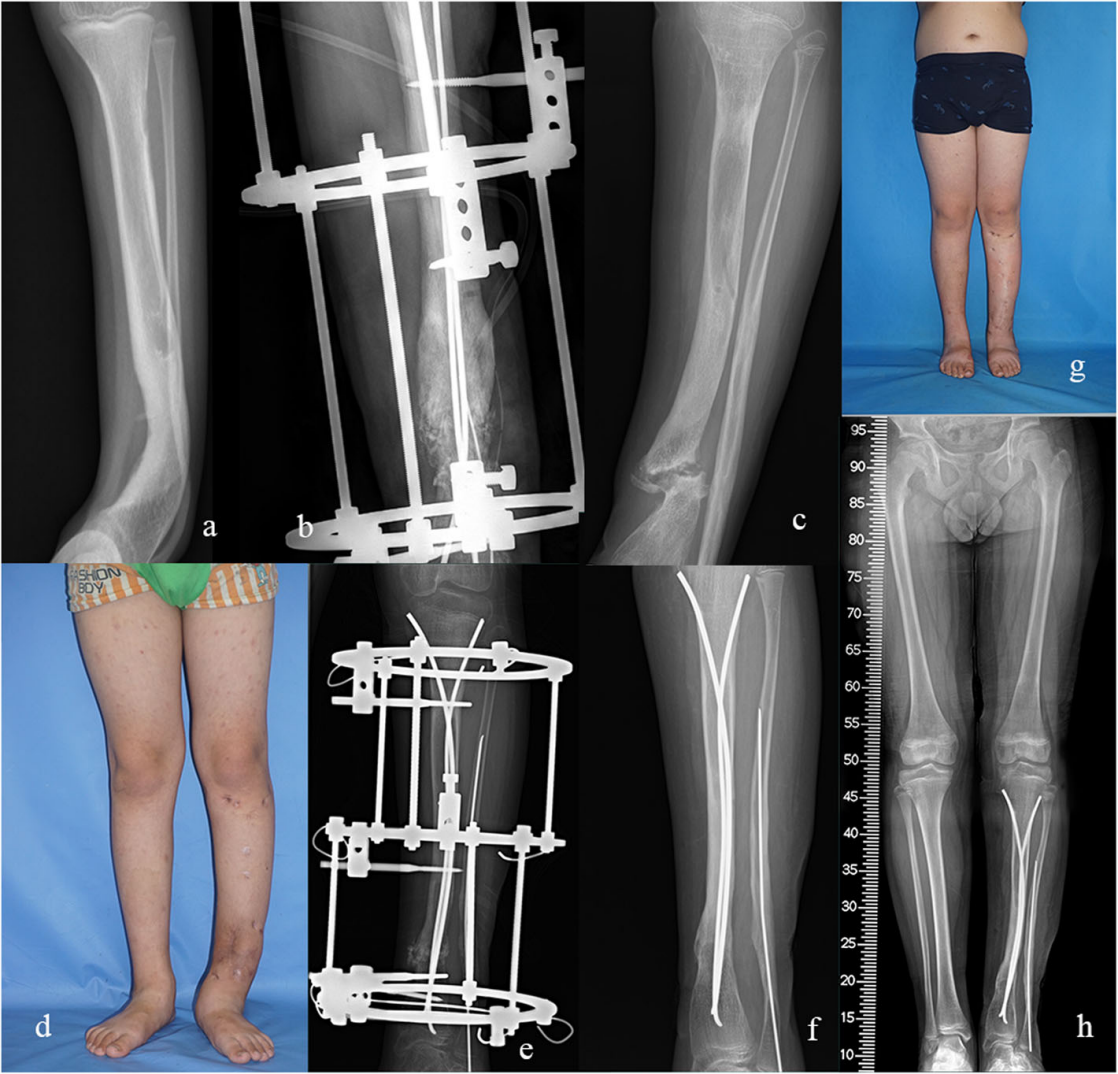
Anteroposterior radiograph of a 7.3-year-old boy with Crawford type II CPT (Case 3) (a). He underwent additional bone grafting and prolonged external fixation due to delayed union after initial combined surgery (b). However, refracture occurred 1 month after removal of Ilizarov fixator. He received cast immobilization and removal of broken nails at local medical center (c, d). In our institute, double TENs insertion and external fixation were applied (e). The fracture healed 5 months later and the external fixator was removed (f). Clinical and radiological appearance shows a well-aligned lower limb and sound union of the pseudarthrosis site (g, h)
